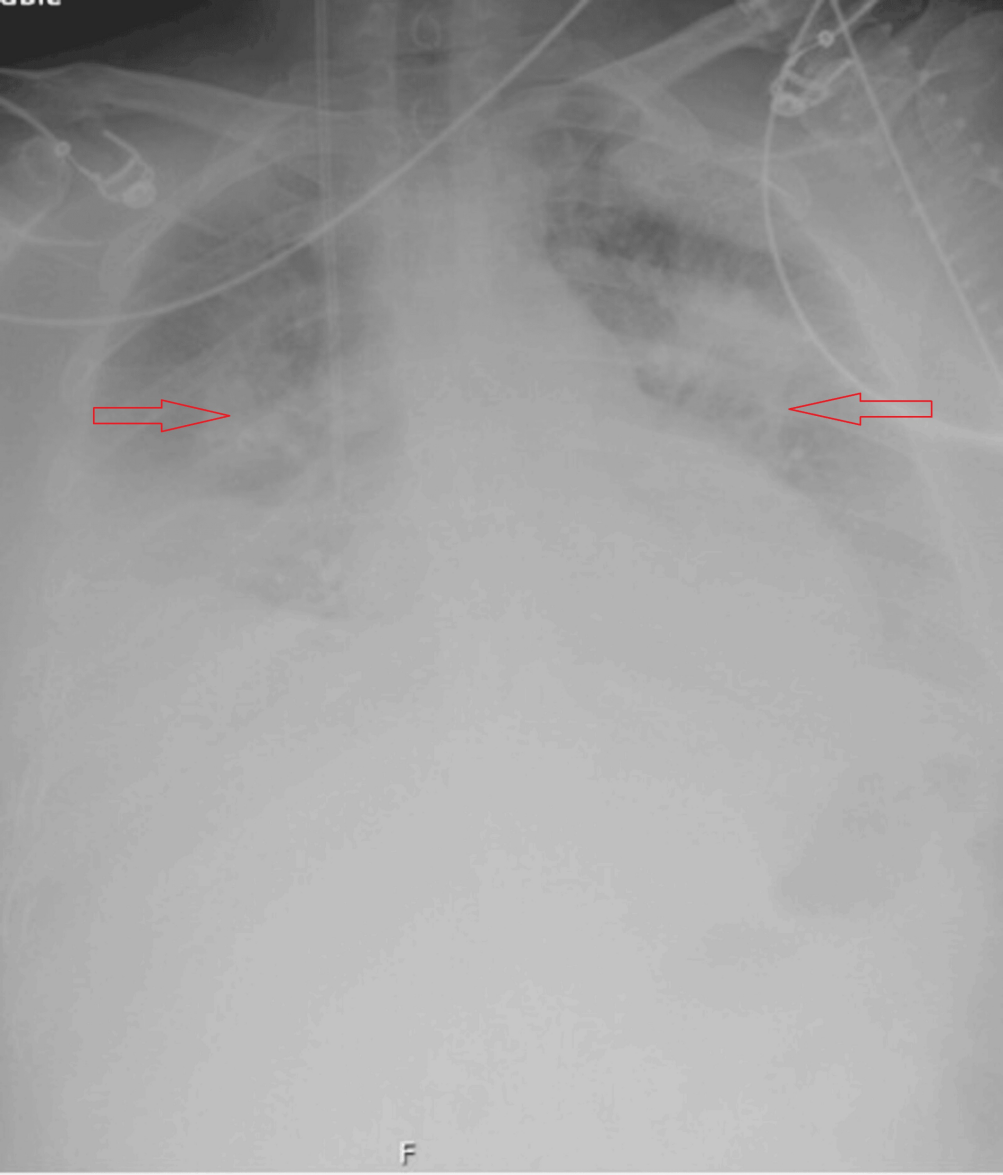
X-ray chest showing evidence of pulmonary congestion following high-dose insulin euglycemic therapy (HIET) in the treatment of calcium channel blocker (CCB) toxicity in the patient
